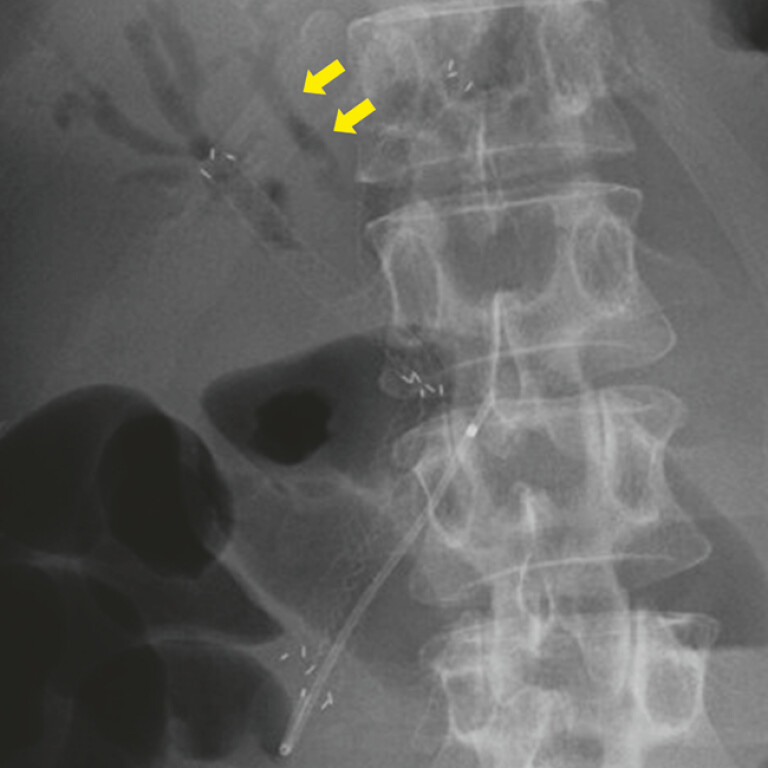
An X-ray image taken the next day reveals the disappearance of the contrast agent. Yellow arrows: pneumobilia in the B4 branch.
